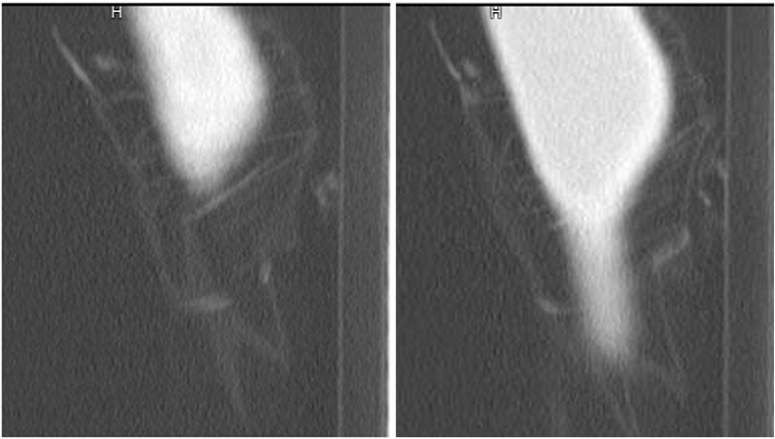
Bird peak appearance characteristic for pseudoachalasia on computed tomography scan.
